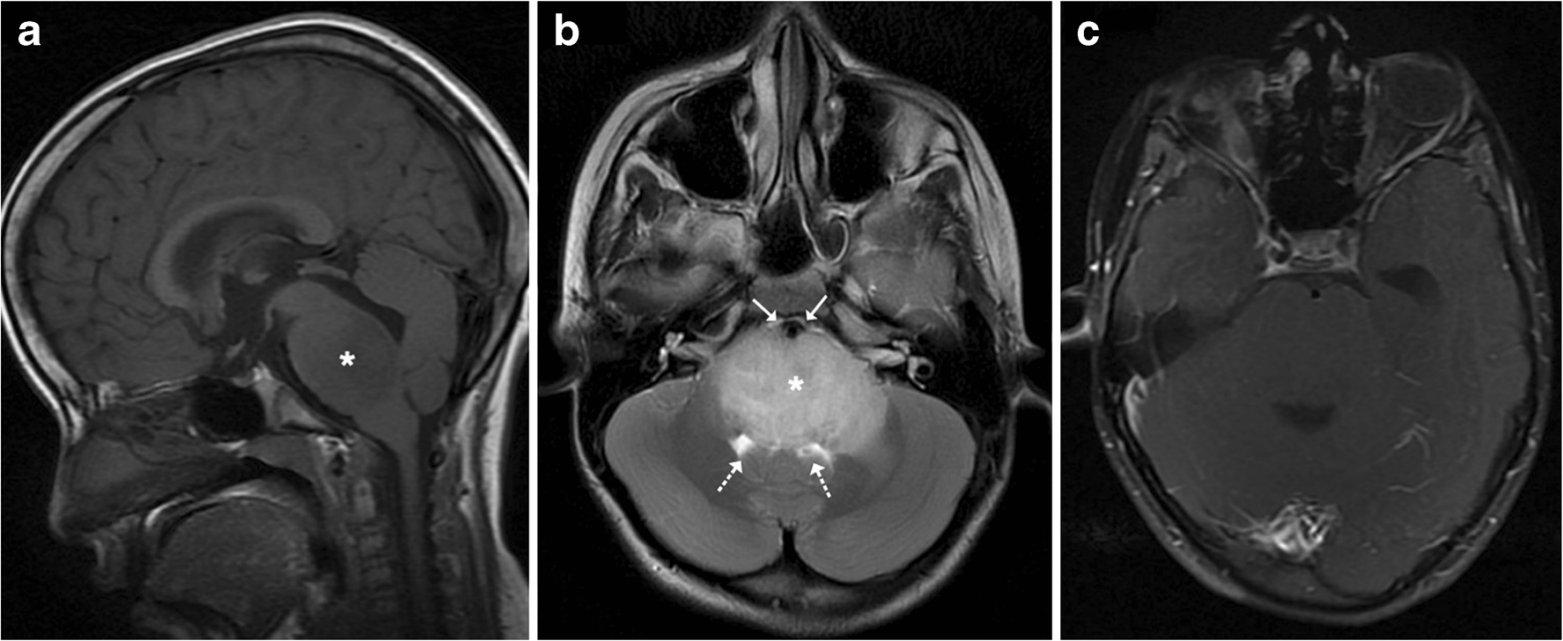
A 9-year-old child with headache and vomiting: a Sagittal T1-weighted image shows expansion of the pons with hypointense signal (*asterisk*). b Axial T2-weighted image shows increased signal intensity involving the pons (*asterisk*) with the basilar artery (*arrow*) appearing engulfed by the lesion. Posteriorly the fourth ventricle is compressed (*dotted arrow*) (flat fourth ventricle sign). c No significant contrast enhancement is noted within the lesion
